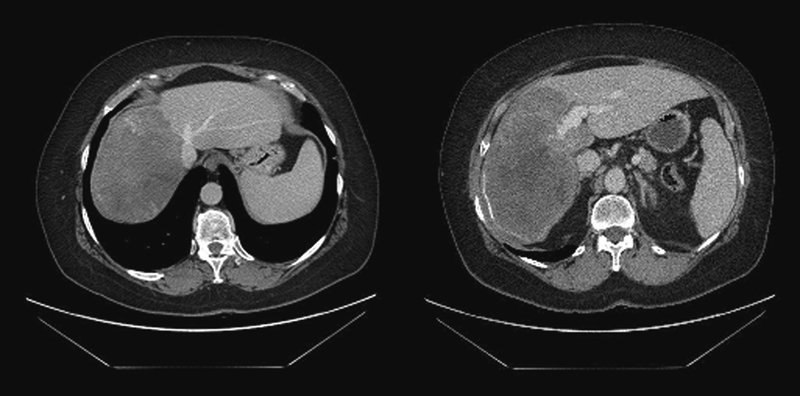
Abdominal computed tomography with contrast media. (Left) A mass with diffuse amorphous calcification in the right liver lobe. (Right) The lesion with calcified margins occupying the right liver lobe and involving the portal vein system.
